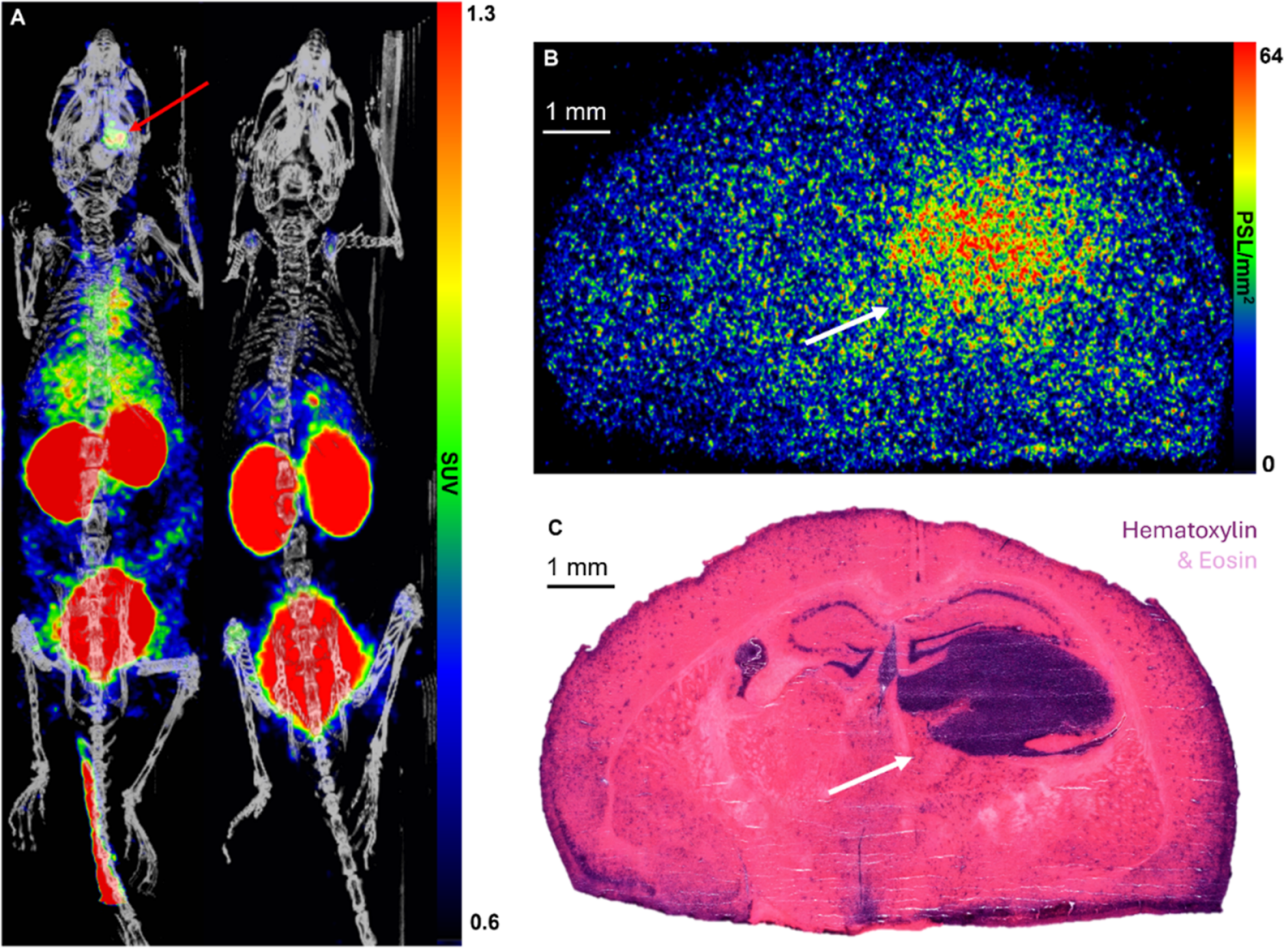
(A) Representative whole-body [^18^F]­FNA PET/CT
